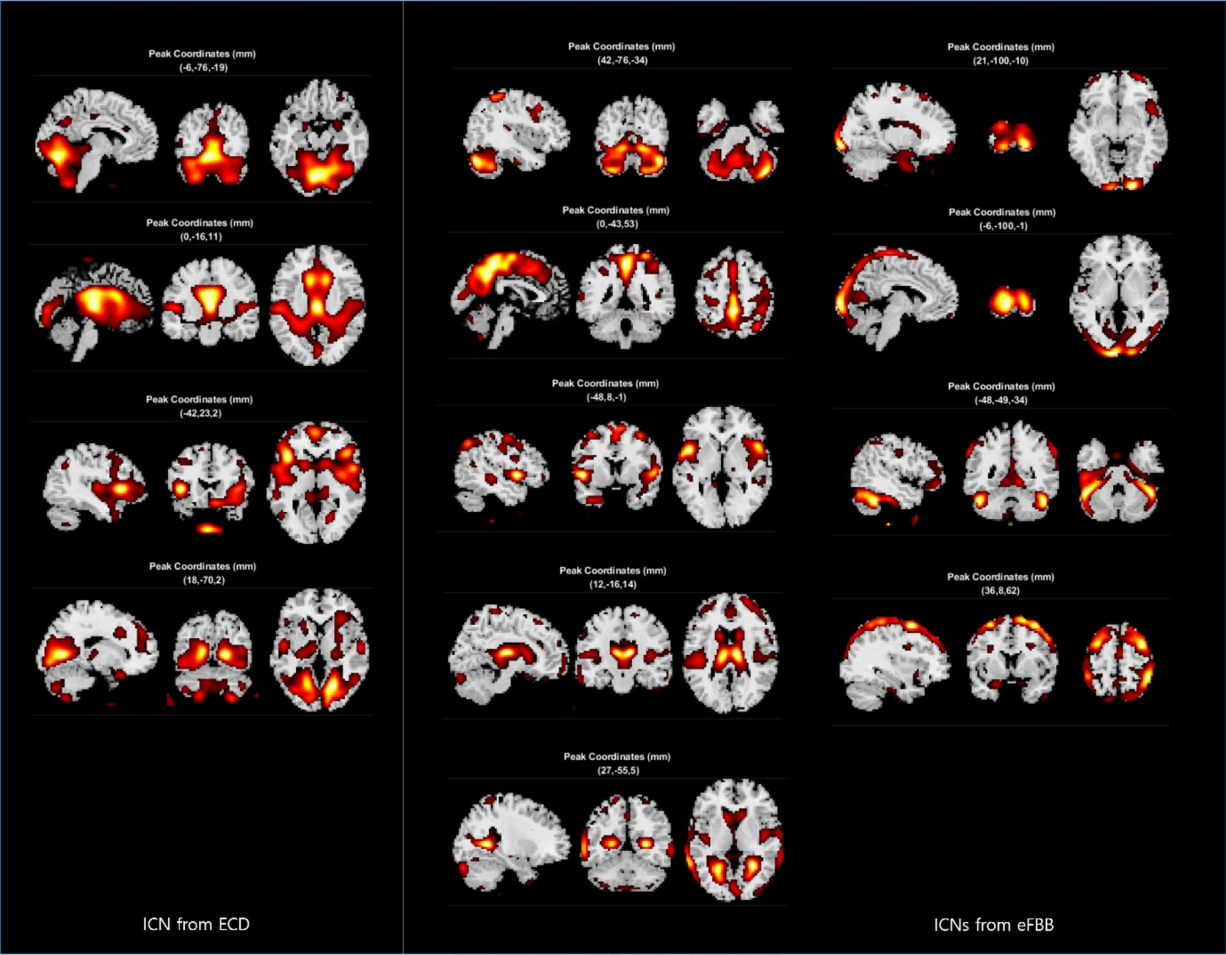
The intrinsic connectivity networks identified from ECD SPECT and eFBB PET. The images in the 1st column show the intrinsic connectivity networks derived from ECD SPECT, and the 2nd and 3rd columns from eFBB PET. Abbreviations ECD SPECT, Tc-99m ethyl cysteinate dimer single photon emission computed tomography; eFBB PET, early-phase F-18 Florbetaben positron emission tomography.
